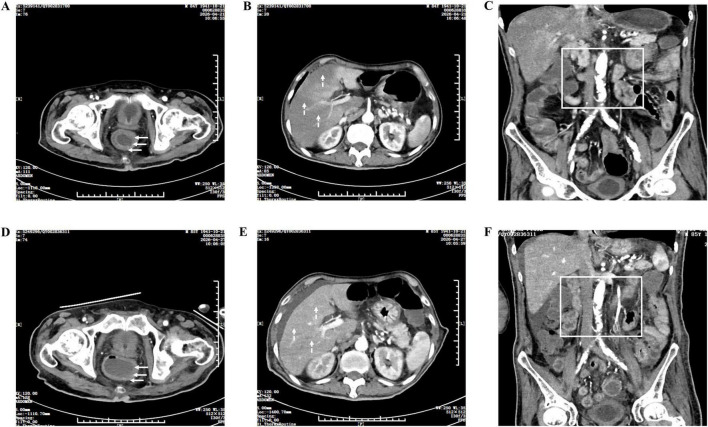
Contrast-enhanced abdominal computed tomography (CT) findings before and after treatment, showing changes in ischemic bowel injury, hepatic hypoperfusion, and penetrating atherosclerotic ulcer of the abdominal aorta. (A,D) Axial contrast-enhanced abdominal CT images before and after treatment, respectively, showing bowel changes. Before treatment, thickening and edema of the rectal/sigmoid colon wall were observed; these findings were alleviated after treatment. Solid white arrows indicate the affected bowel wall. (B,E) Axial contrast-enhanced abdominal CT images before and after treatment, respectively, showing hepatic perfusion changes. Before treatment, multiple patchy hypoenhancing areas were observed in the liver parenchyma, suggesting hepatic hypoperfusion/ischemia; hepatic enhancement improved after treatment. Dashed white arrows indicate the areas of hypoperfusion. (C,F) Coronal contrast-enhanced CT images before and after treatment, respectively, showing abdominal aortic lesions. A focal contrast-filled outpouching was observed in the abdominal aortic wall, consistent with mural thrombus and formation of a penetrating atherosclerotic ulcer. The lesion remained morphologically stable after treatment, without obvious progression. The white boxes indicate the PAU region, located in the infrarenal abdominal aorta.
